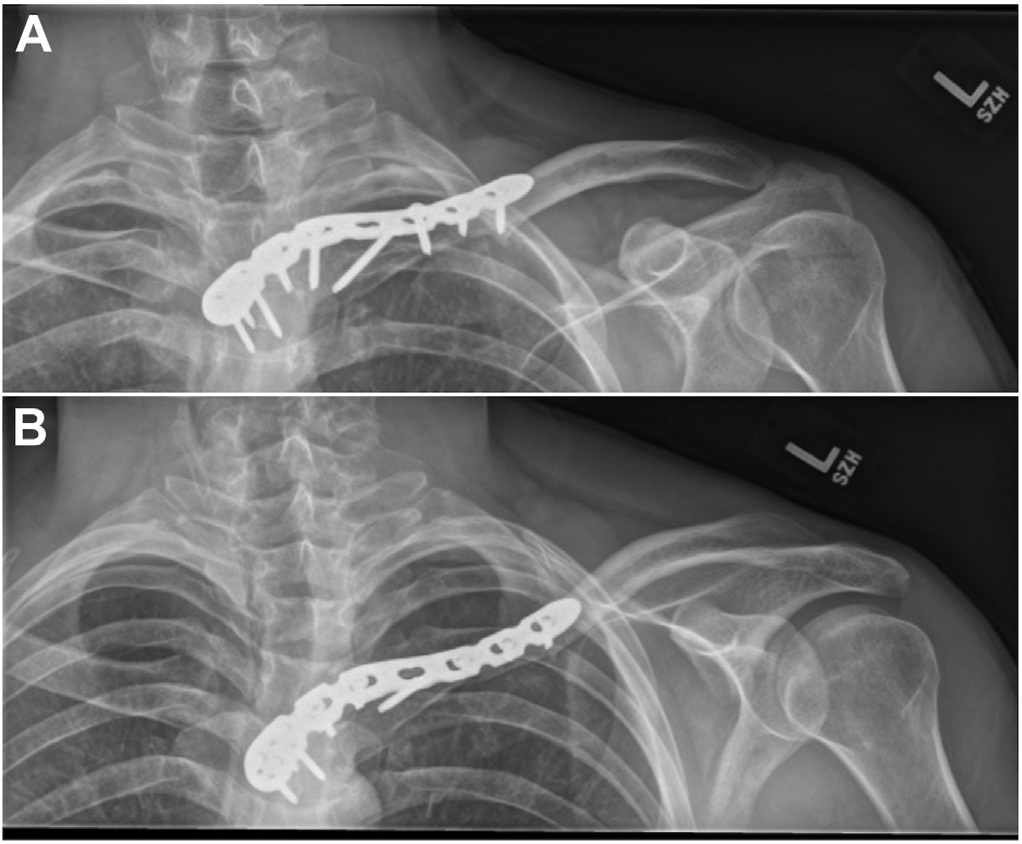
Postoperative radiographic imaging, including (A) anteroposterior view of the clavicle with cephalic angulation and (B) anteroposterior view of the left clavicle, shows fracture reduction and hardware positioning.
